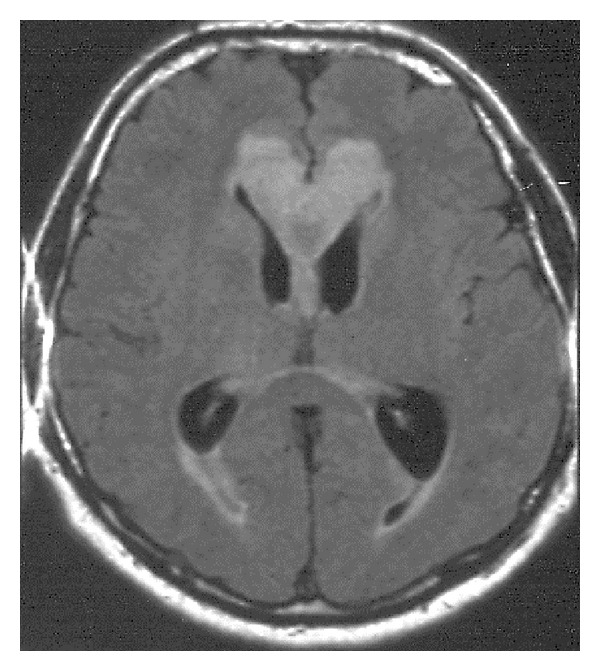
FLAIR, axial plane. Lymphoma affecting callosal genu.
